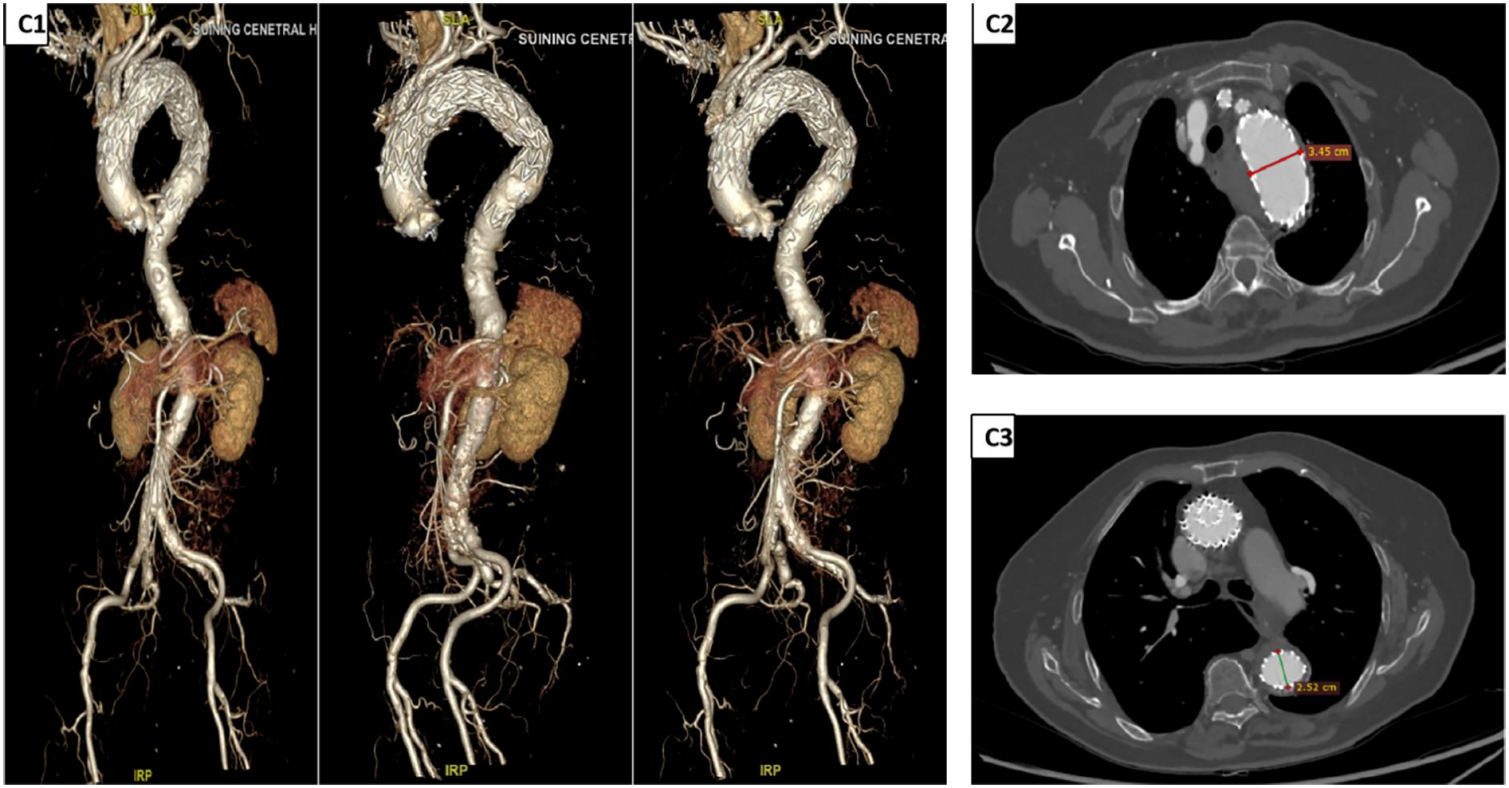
Postoperative DSA and CTA findings. (C1) DSA images at different time points after TEVAR (from left to right: 1 week, 1 month, and 6 months post-operation) show that the stent position remains stable, with no evidence of endoleaks. The vascular morphology and hemodynamics are stable. (C2,C3) CTA images 1 week after TEVAR. Measurement results indicate that the diameter of the surgical area in the aortic arch is approximately 3.45 cm, and the diameter of the area covered by the TEVAR procedure is approximately 2.52 cm, suggesting a good effect of aneurysm exclusion and a normal process of vascular remodeling. DSA, digital subtraction angiography; CTA, computed tomography angiography; TEVAR, total endovascular aortic repair.
